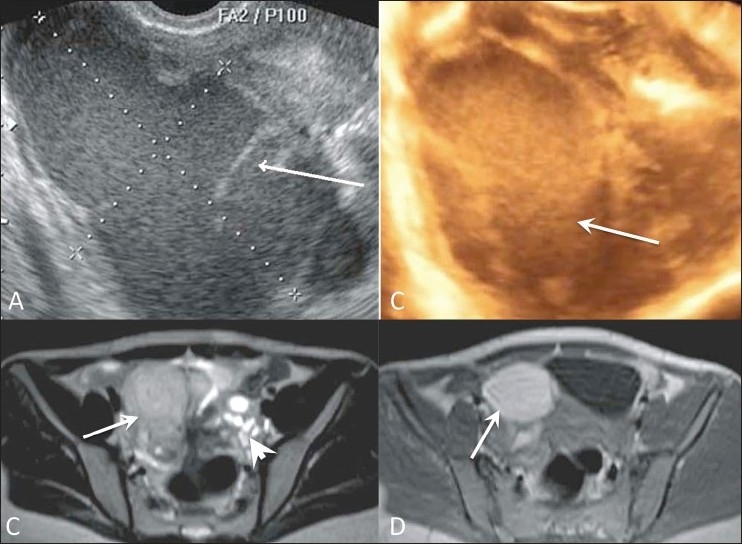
Endometrioma. Transvaginal gray-scale (A), 3D color USG (B) USG, axial T2W (C) and T1W (D) MRI images show an endometrioma (arrows) on the right side and a polycystic ovary (arrowhead) on the left side in two different patients. Note the thick septae in the endometrioma (arrow in A)
